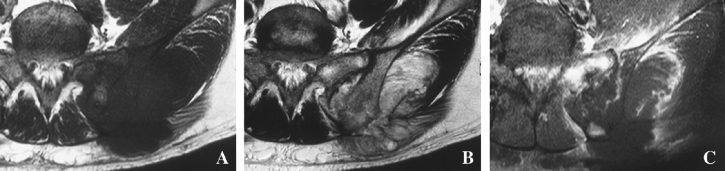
Case 2. (A) MRI showed a low-intense lesion around the left sacroiliac joint on T1-weighted images. (B) T2-weighed images confirmed a heterogenous hyperintense lesion. (C) The lesion was enhanced on fat-suppressed T1-weighed images.
